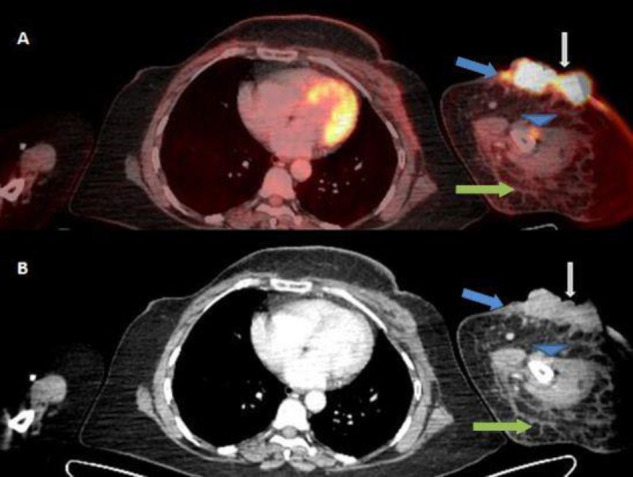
Corresponding fused axial A. FDG PET/CT and B. CECT images show FDG avid (SUV_max_ 20.8) heterogenous enhancing slightly exophytic cutaneous lesion in antero lateral aspect of left arm ( white arrow), with adjacent cutaneous thickening( SUV_max_ 7.8) ( blue arrow), edema and stranding of the subcutaneous fat (green
